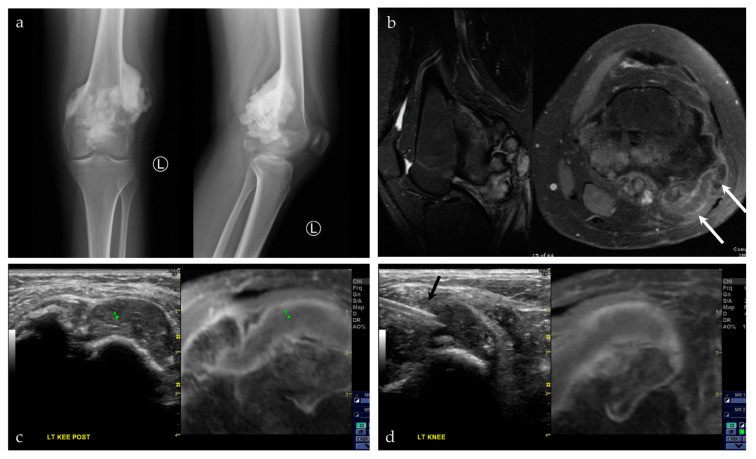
A 17-year-old female with a posterior knee mass. From the history, the patient reported a prior tumor removal at an outside hospital, possibly an osteochondroma. (a) AP and lateral radiographs of the left knee show a large, lobulated and densely mineralized mass arising from the posterior surface of the distal femur. Radiographic features are classic for parosteal osteosarcoma. (b) Sagittal T2 fat saturated sequence (left) shows multiple mineralized lobules at the posterior knee, with areas of high T2 signal interspersed between these lobules; axial post-T1 fat saturated sequence (right) shows patchy areas of enhancement around this densely mineralized mass. There is also a cartilage cap, seen at the lateral aspect of the lesion (white arrows). (c,d) Captured images from a US-MRI guided fusion. The black arrow shows the biopsy needle within the cartilage cap portion of the lesion. This was the requested area for biopsy. The preliminary biopsy report was a chondroblastic lesion, without further characterization. Upon complete resection of the lesion, the final pathology was parosteal osteosarcoma with a prominent chondroblastic component. The final pathology result is consistent with the radiographic features of the lesion.
